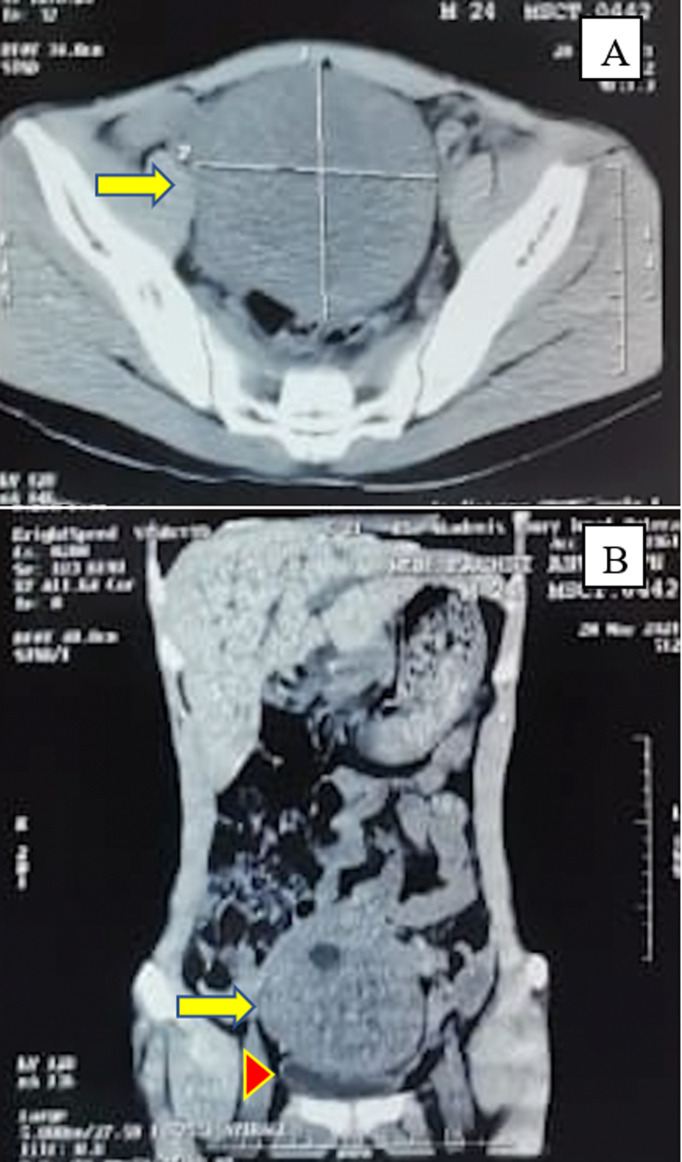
an abdominal computed tomography scan without contrast showing an intra-abdominal mass lesion (yellow arrow) pushing the bladder caudally (red arrowhead) sized 13 x 9 cm; A) axial view; B) coronal view
